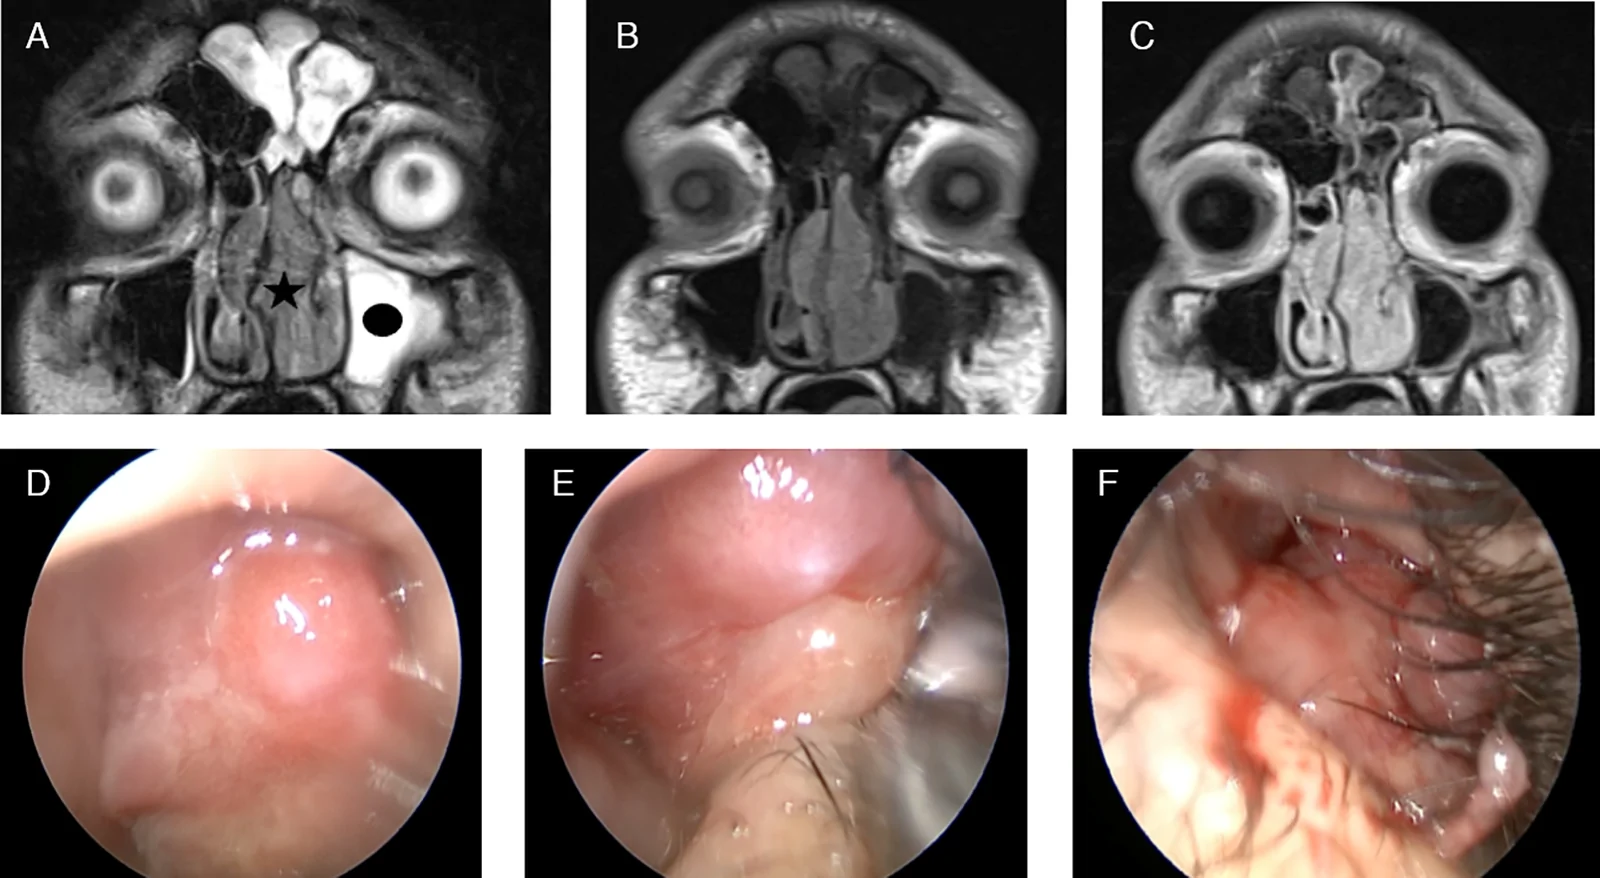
Radiologic and endoscopic findings in nasal rhinoscleroma: (A–C) Axial magnetic resonance imaging (MRI) scans (T2-weighted in A, T1-weighted in B, and contrast enhanced T1-weighted in C) of the nasal cavity and paranasal sinuses showing an infiltrative soft-tissue lesion involving the nasal septum and adjacent nasal structures, consistent with a chronic granulomatous process, without evidence of bone destruction. (D–F) Endoscopic views of the nasal cavity demonstrating an irregular, polypoid mucosal mass with friable surface and inflammatory appearance.
